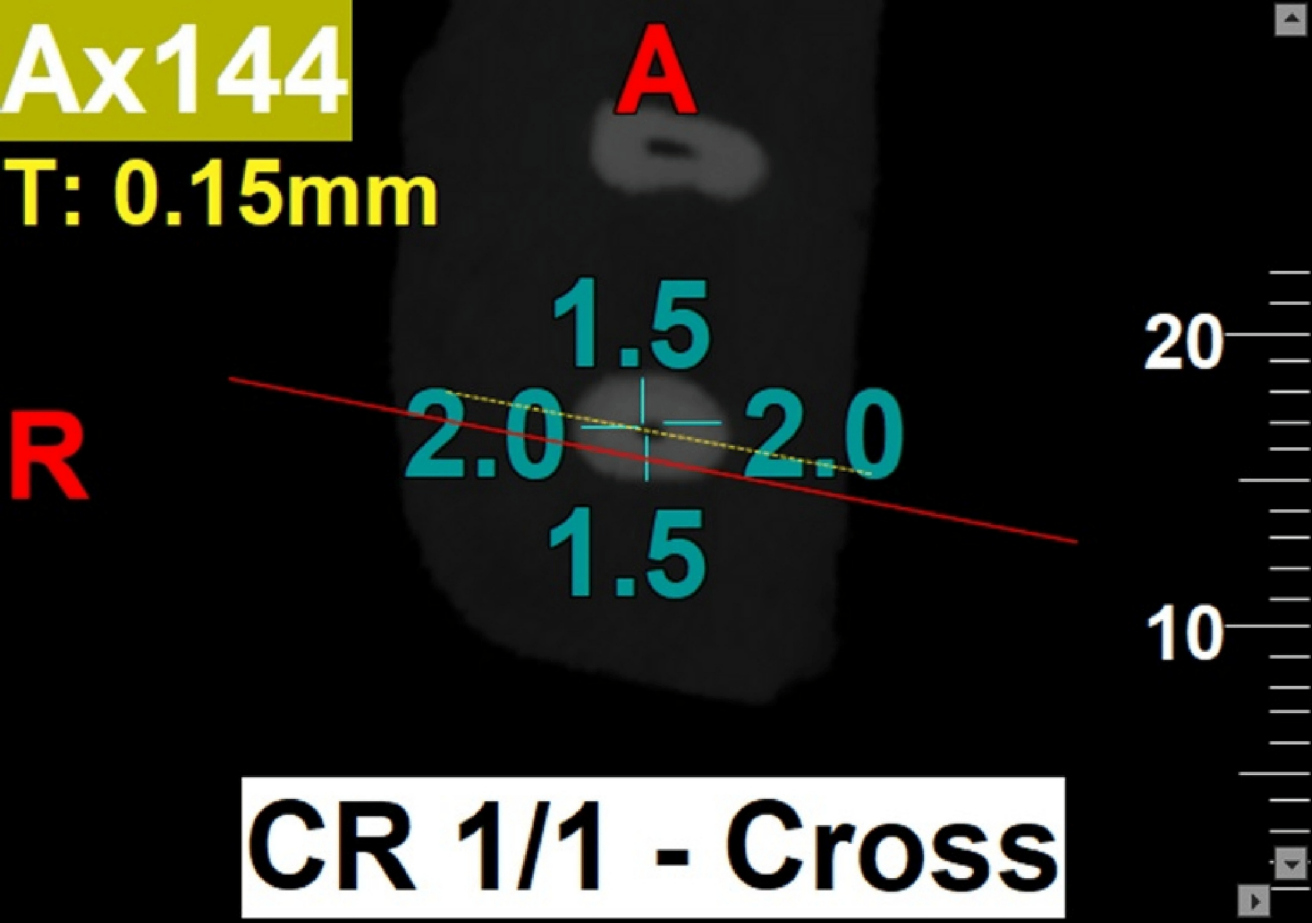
Post-instrumentation CBCT at 3 mm from pre-determined working length. CBCT: Cone beam computed tomography
